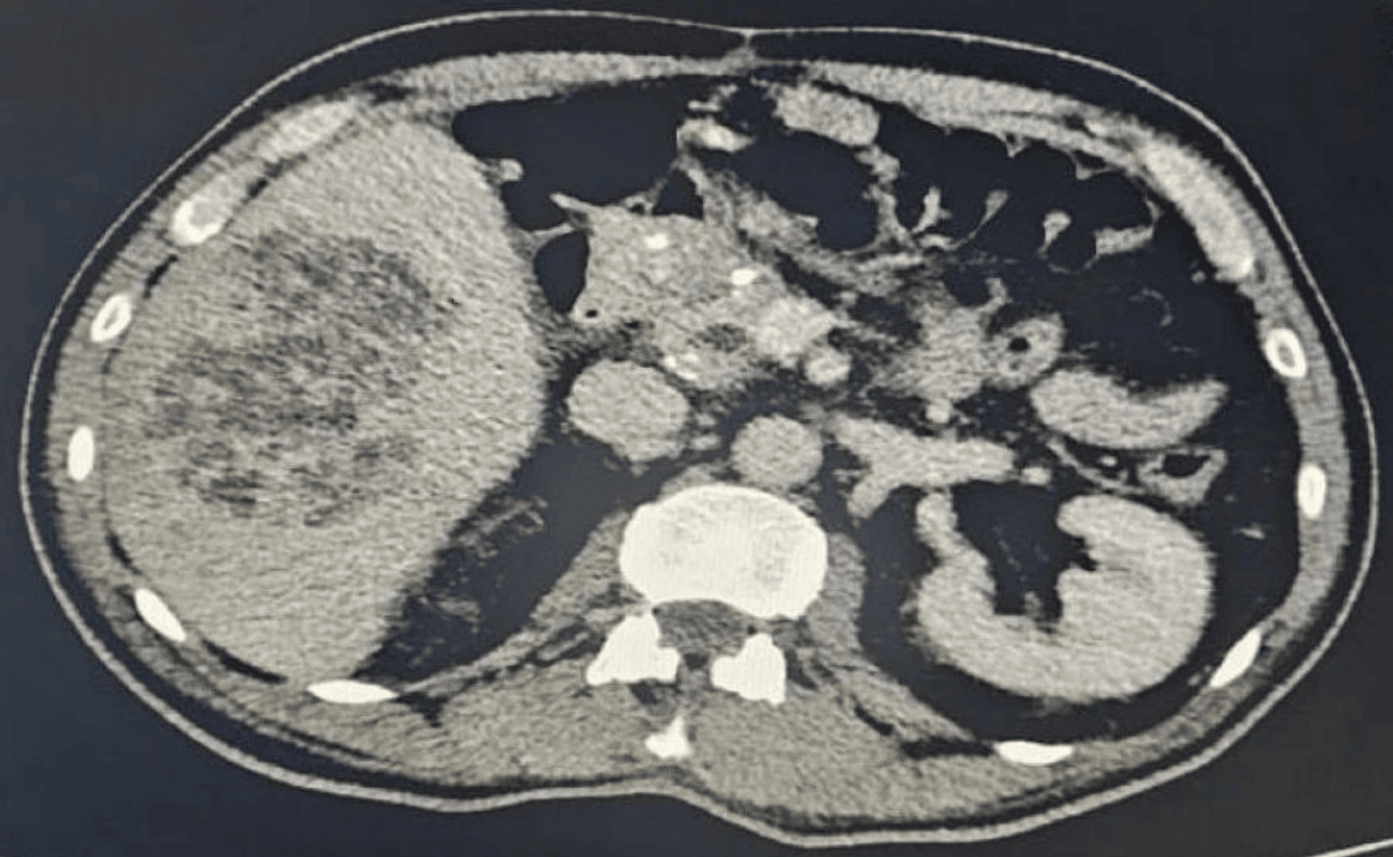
Contrast-enhanced CT showed a large septated mass in the right hepatic lobe (88 x 61 x 84 mm) and an adjacent mass (36 x 26 x 39 mm) with a bulky pancreatic head and dilated pancreatic duct (9 mm)
